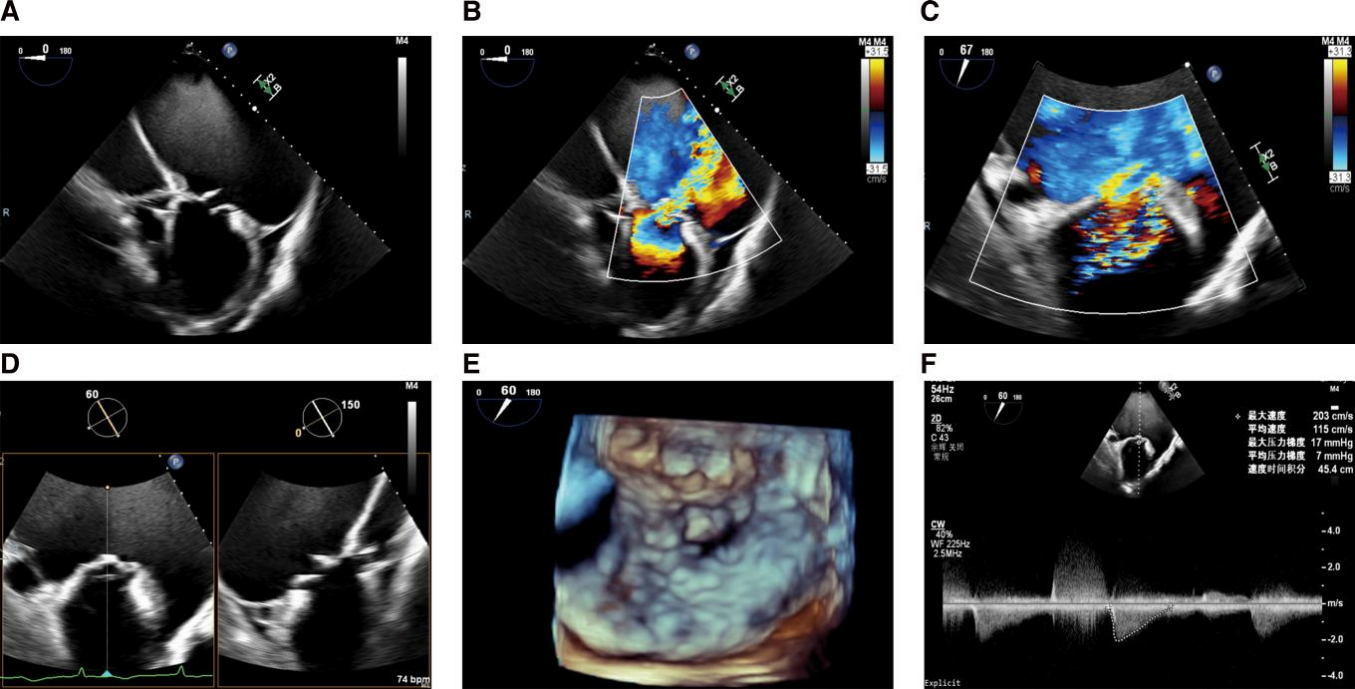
Preprocedural transoesophageal echocardiography. (*A–F*) Preprocedural assessment demonstrating severe mitral regurgitation due to anterior and posterior leaflet prolapse at the A2–P2 segment.
